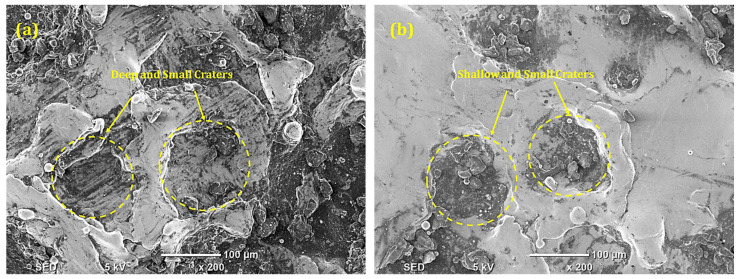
SEM of machined surface in Kerosene-S-20 modified dielectric using (a) CT Cu electrode; (b) CT brass electrode.
